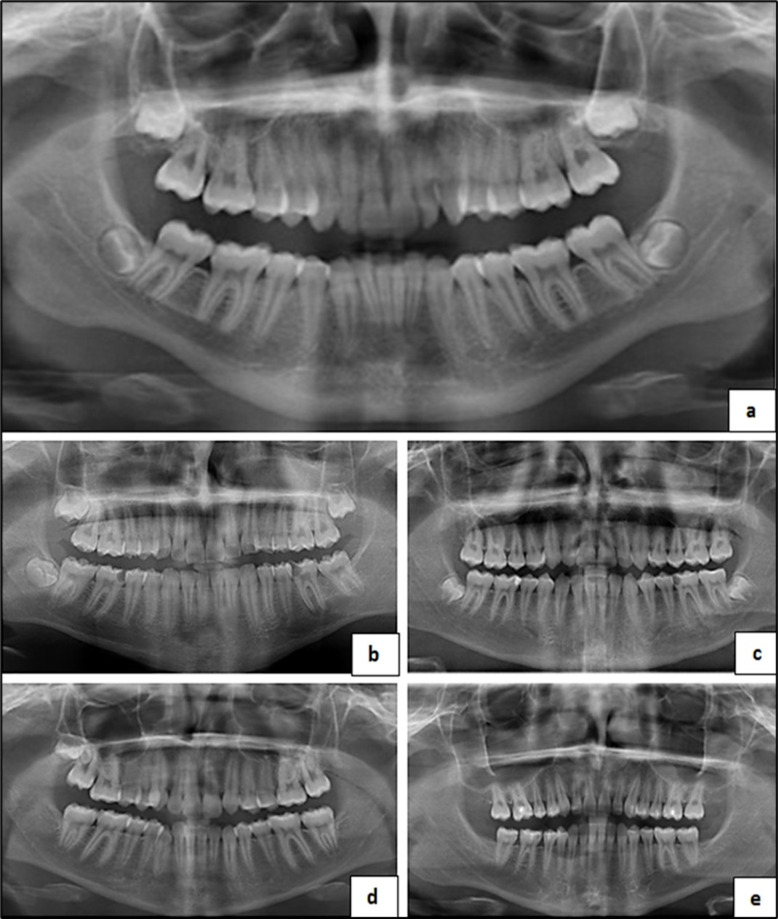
Panoramic radiographs showing third-molar agenesis patterns: (a) all third-molar germs present, (b) single agenesis, (c) double agenesis, (d) triple agenesis and (e) total agenesis.
